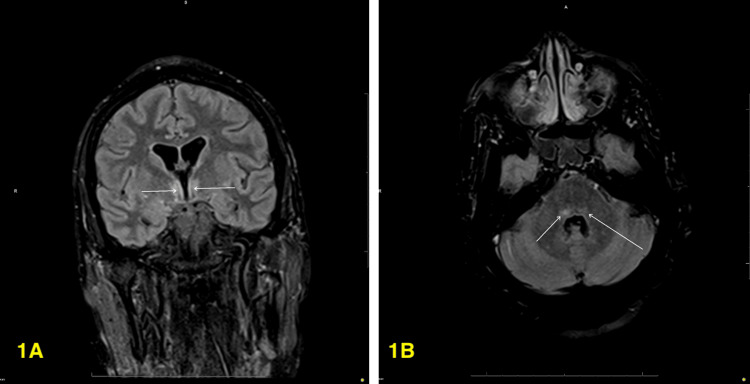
Brain fluid-attenuated inversion recovery (FLAIR) MRI. (A) Coronal T2/FLAIR images demonstrate an abnormal, symmetric hyperintense signal involving the columns of the fornices. (B) Axial T2/FLAIR images demonstrate an abnormal hyperintense signal involving the right greater than left dorsal pons.
